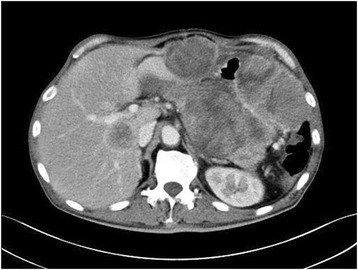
Contrast-enhanced CT at recurrence on postoperative day 36, showing peritoneal dissemination and liver metastases
